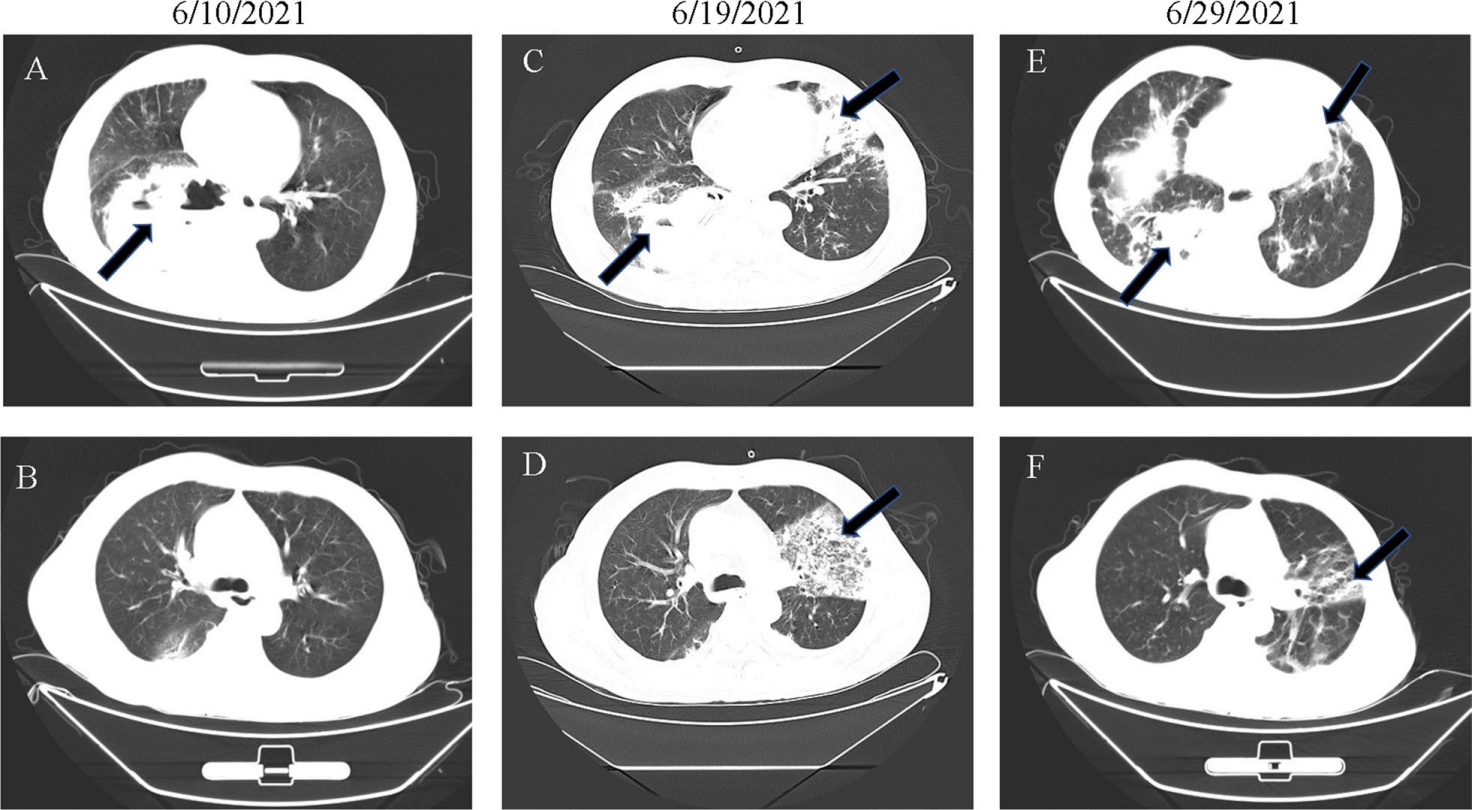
Computed tomography (CT) results of the *Olsenella uli*-induced pneumonia. Considering the day when the patient was transferred to our hospital as the first day, A, B CT on the second day(6/10/2021) showed bilateral pneumonia, pyogenic necrosis in the right lower lobe of the lung, encapsulated pyothorax in the right chest and bilateral emphysema, as the arrow indicated; C, D CT on the tenth day(6/19/2021), the arrows showed worsening bilateral pneumonia and emerging bilateral pleural effusion compared with the second day; E, F CT on the twentieth day (6/29/2021), arrows showed improvement in the bilateral pneumonia and left pleural effusion in comparison with the tenth day
